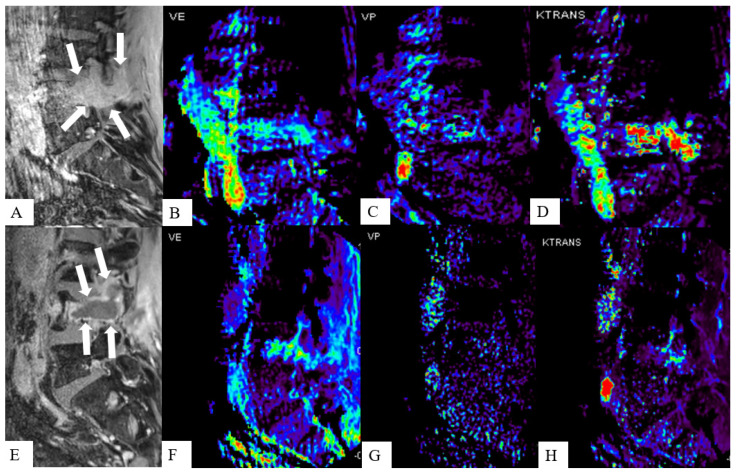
Monitoring response treatment in a 51-year-old male with spine metastasis from renal cell carcinoma. Pre-SBRT images (A–D) show a solid lesion with irregular margins and moderate enhancement on the sagittal post-contrast T1-weighted image (A) involving the vertebral body and pedicle (arrows). Ve (B), Vp (C), and K-trans (D) dynamic contrast-enhanced parametric maps show increased perfusion indices within the lesion. Post-SBRT imaging (E–H) shows considerable central necrotic change in the mentioned tumor 4 months after SBRT treatment (arrows). Notice the significant lesion perfusion loss depicted in Ve (B), Vp (C), and K-trans (D) parametric maps indicating treatment response, despite stable lesion size on conventional MRI, highlighting the ability of DCE perfusion to better represent metastatic lesion biological activity.
